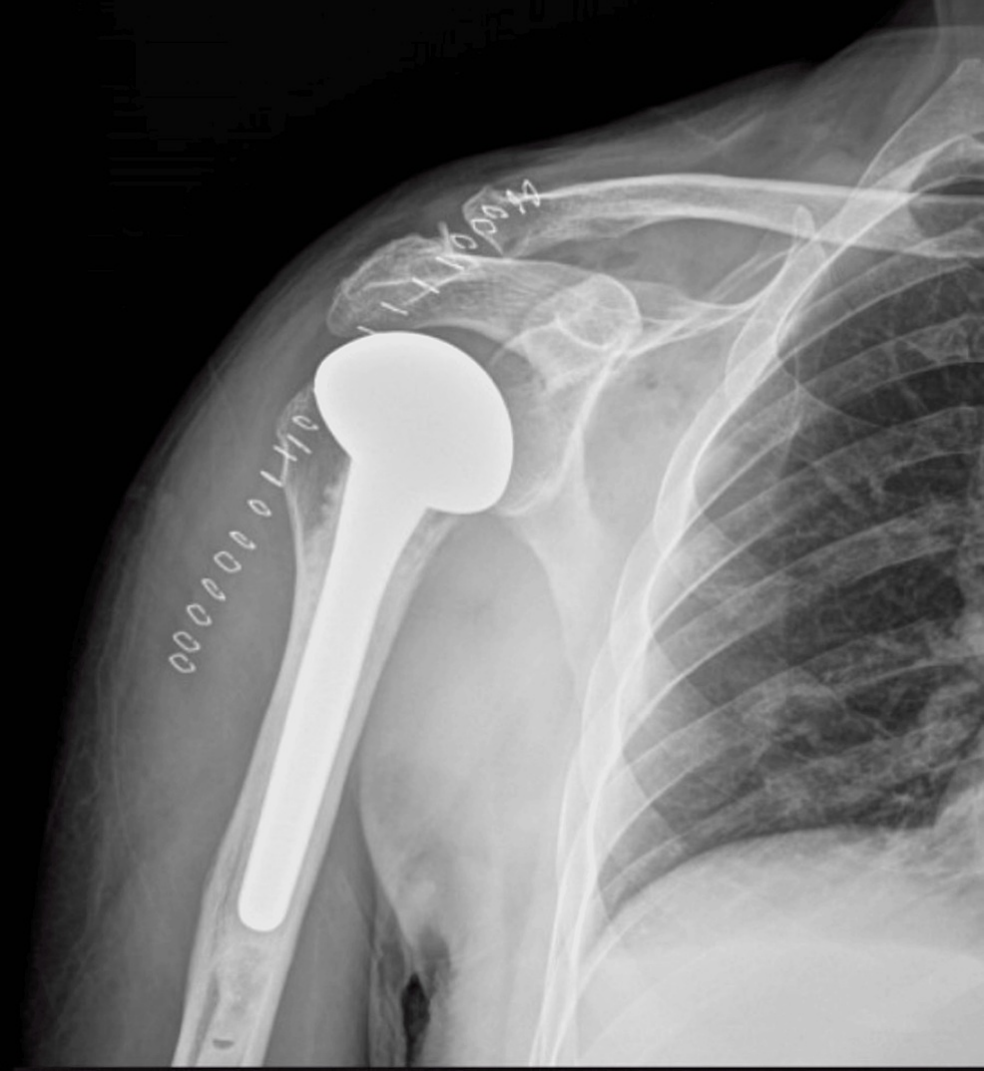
Postoperative anteroposterior x-ray of the right shoulder. The patient has been treated with hemiarthroplasty.
